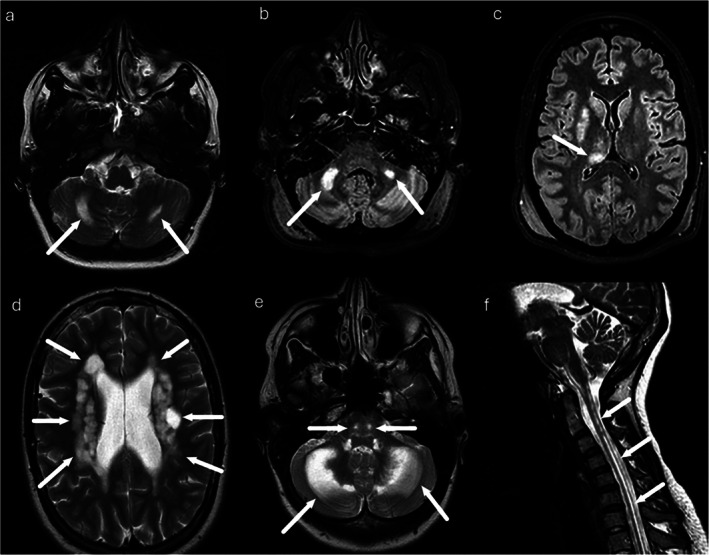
Brain MRI findings related to patients with *POLG*‐related disease and LBSL. (a–c) *POLG*‐related disease in a patient imaged first in 2007 (a; age 22, T2‐weighted) and again in 2014 (b, c; age 29, FLAIR). (a) Bilateral cerebellar WMH were already present years before the first SLE and remained radiologically stable. (b, c) Follow‐up imaging shows persistence of supratentorial WM lesions with the appearance of new abnormalities in the right thalamic region. (d–f) LBSL findings in 23‐year‐old patients (T2‐weighted). Demonstrating the characteristic extensive supra‐ and infratentorial white matter abnormalities, including involvement of the cerebellar white matter, dorsal brainstem pathways, deep nuclear structures and the cervical spinal cord.
